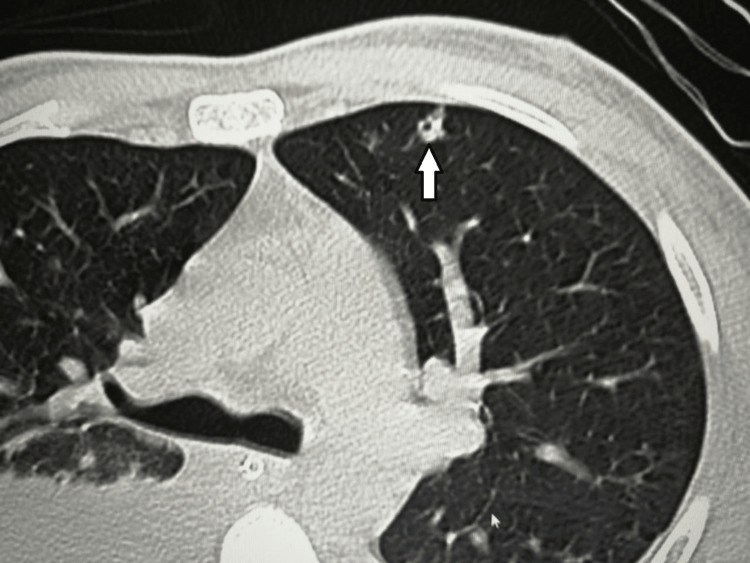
A CT scan of the chest The scan shows a small cavitary nodule in the anterior segment of the left upper lobe (white arrow).
